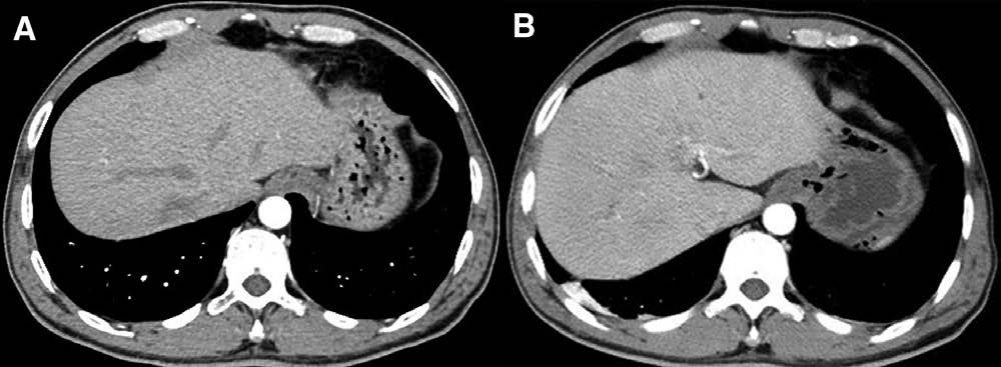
CT scan. (A) CT revealed normal lower lobe of the both lungs before the first P-ESWL. (B) Chest CT showed slight pleural effusion after the second P-ESWL. CT = computed tomography, P-ESWL = pancreatic extracorporeal shock wave lithotripsy.
